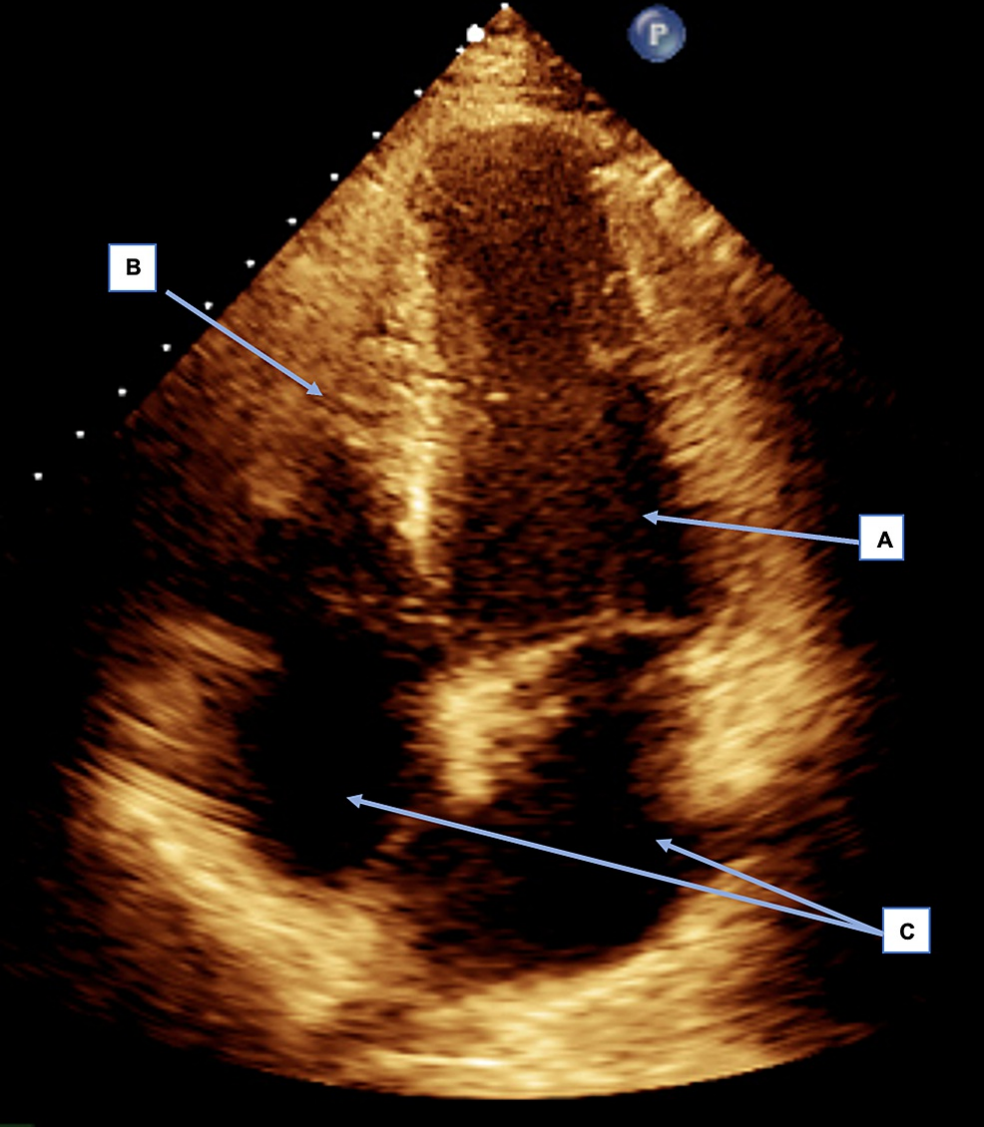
Pre-treatment TTE: Apical 4 chamber (A4C) view in systole showing reduced LV systolic function. A: Left ventricle visually appears dilated in this still image suggestive of impaired function in systole; B: The right ventricle visually appears to be contracting maximally in this still image, suggestive of normal function in systole; C: Both atria appear to be normal in size in this still image. TTE: Transthoracic echocardiogram; LV: Left ventricular.
